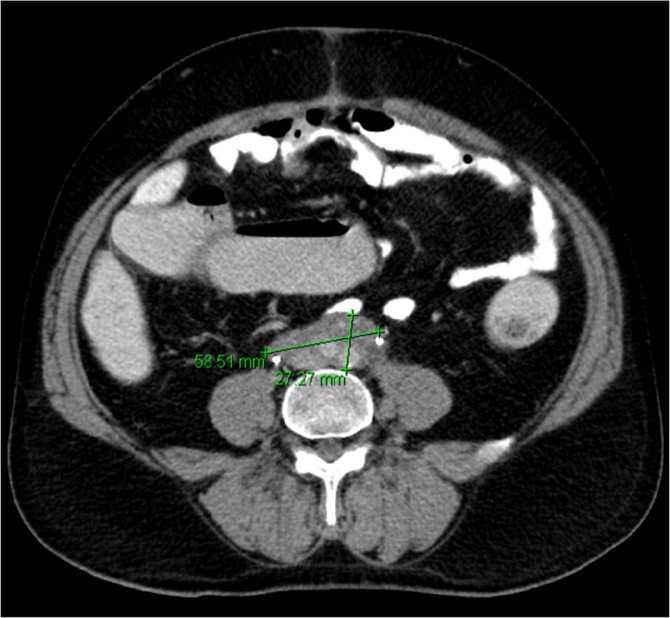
A follow-up CT abdomen that revealed improvement and reduction of retroperitoneal mass.
